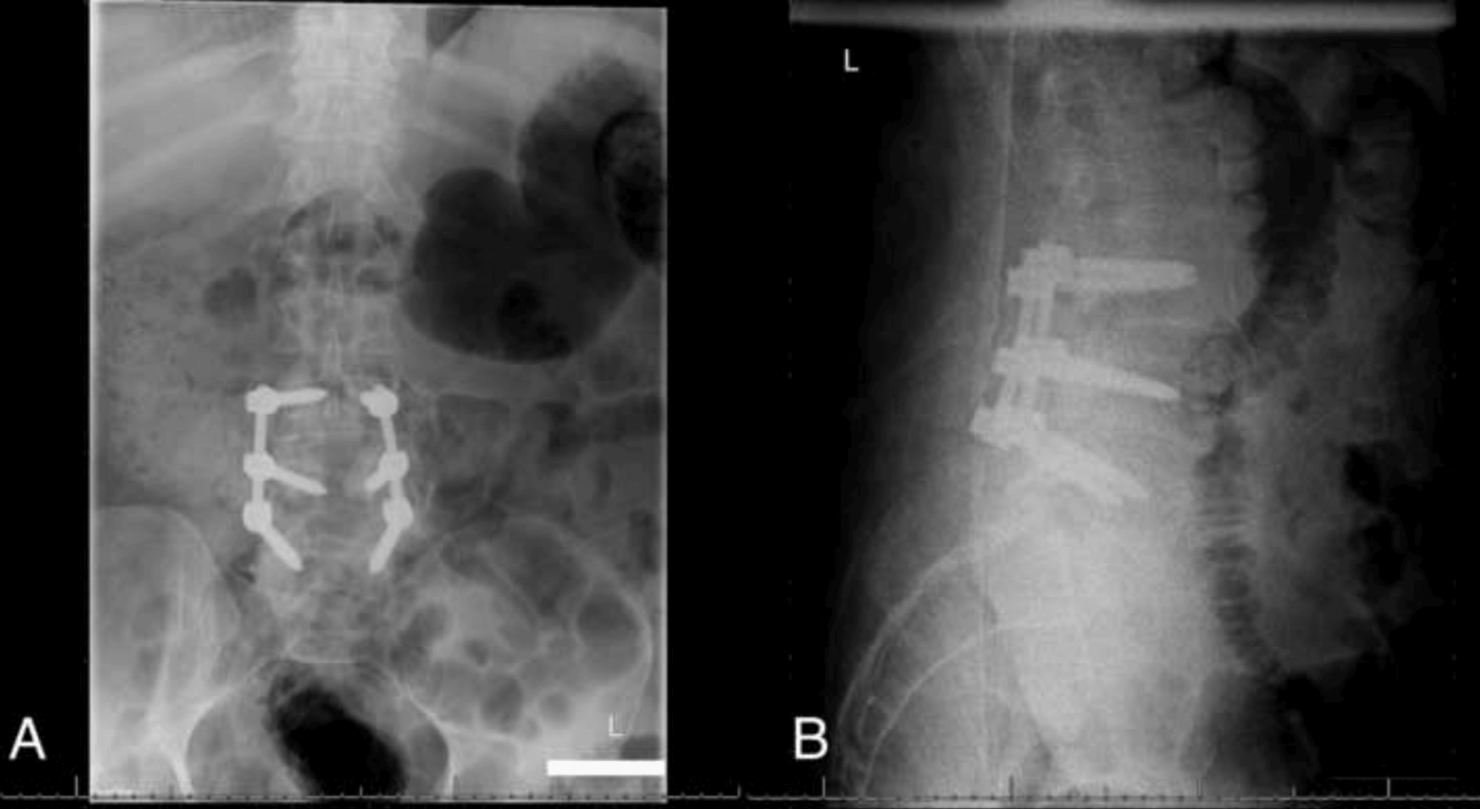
(A) Anteroposterior and (B) lateral radiograph of the lumbar spine demonstrating proper instrumentation alignment of L3-L5 posterior instrumentation.
